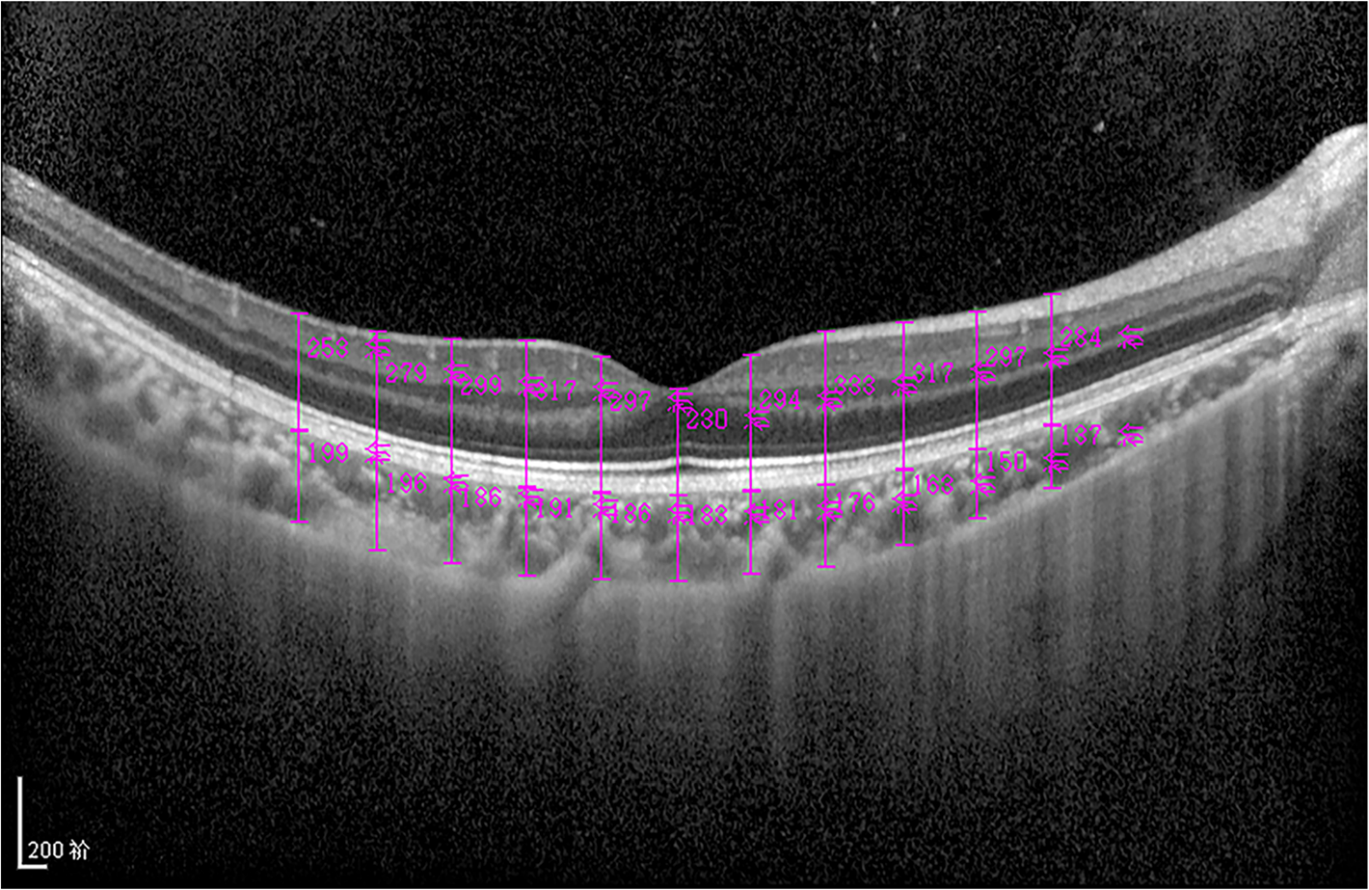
Measurement of RT and CT. RT was measured manually from the inner boundary of the retina to the outer boundary of the RPE, and CT was measured from the outer boundary of the RPE to the inner boundary of the sclera at 0.5-mm intervals along a horizontal line through the macular fovea beginning at the position 2.5 mm to the temporal side of the fovea to the position 2.5 mm to the nasal side of the fovea
